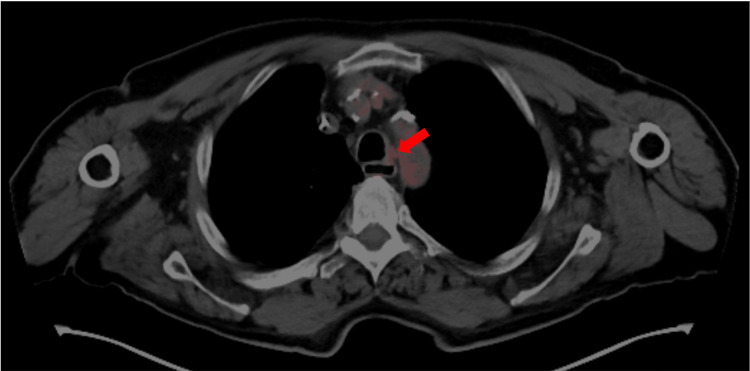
Follow-up PET-CT scan with a red arrow showing a decreased size and FDG uptake at the upper endotracheal lesion PET-CT: Positron Emission Tomography-Computed Tomography; FDG: Fludeoxyglucose
